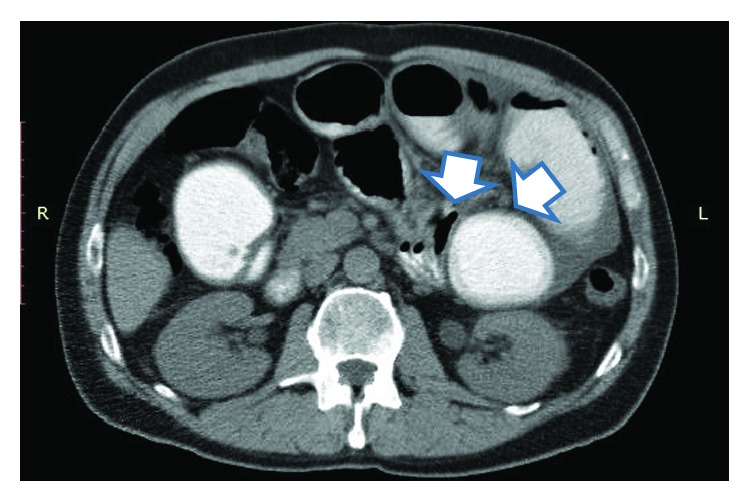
Computed tomography findings of case 2. Dilated small bowel loops, wall thickness, amorphous calcifications, and signs of obstruction could be seen (arrows).
